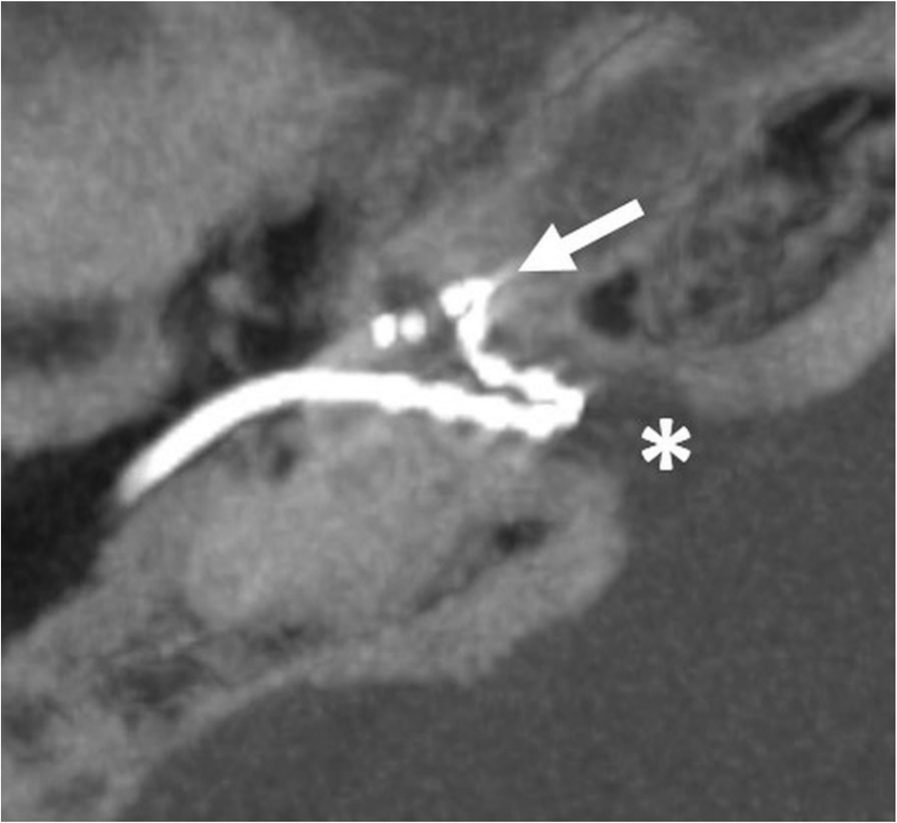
A 20-year-old male patient, with incomplete partition type III and malposition of the electrode in the internal auditory canal. CBCT paraaxial maximum intensity projection image shows part of the electrode array in the basal turn (arrow), but the rest of the electrode forming a slope within the internal auditory canal (asterisk)
